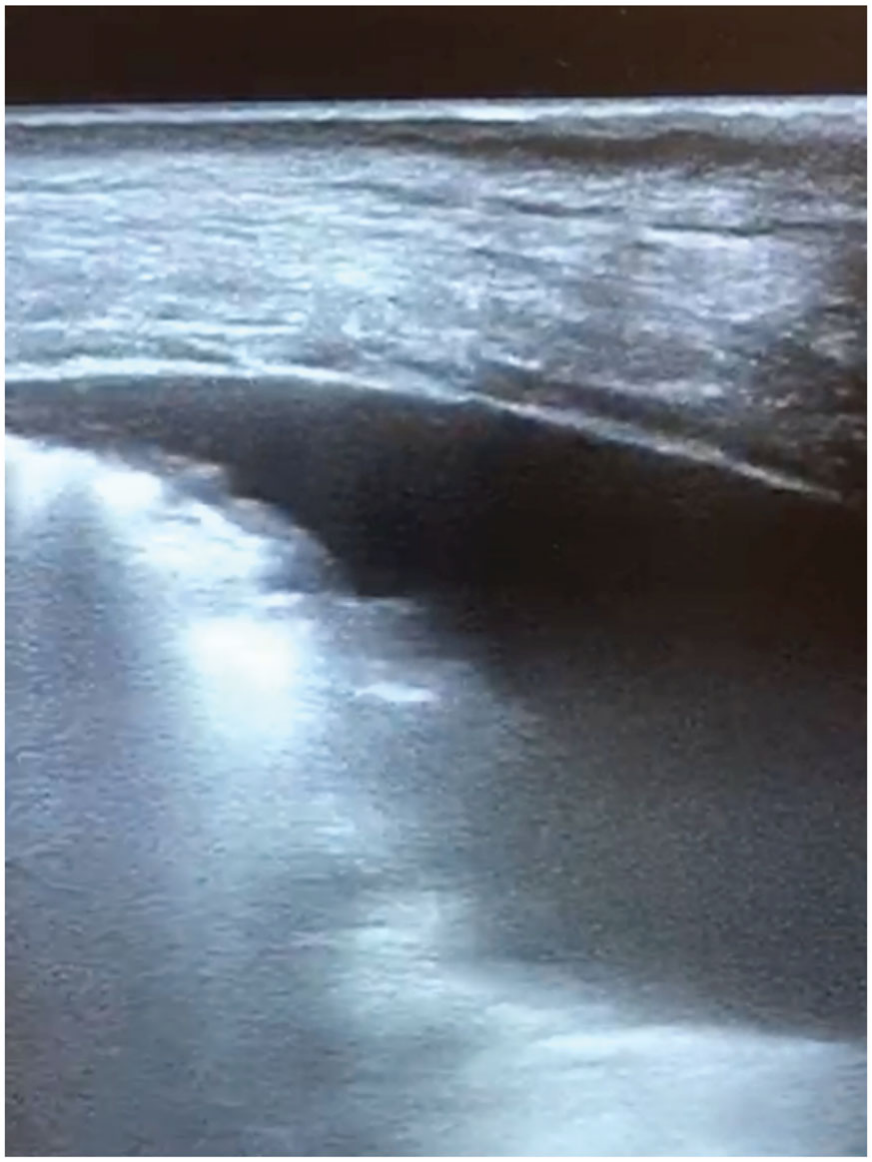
Pleural effusion at lung ultrasound in a SARS-CoV-2 patient appears as an anechoic area (on the right region of the picture).
